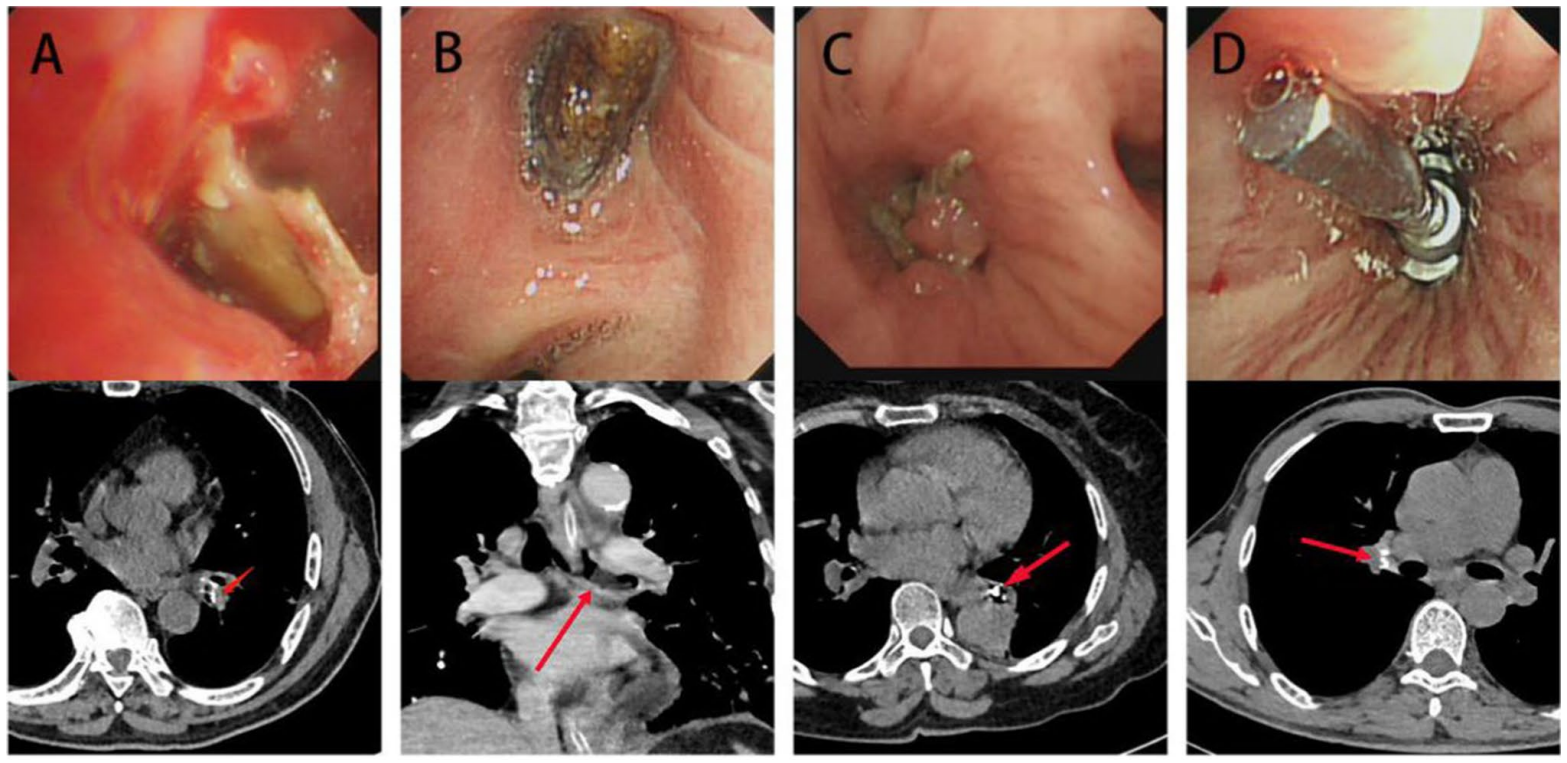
CT and bronchoscopy characteristics of different airway FBs. (A) A bone-like FB in the right inferior lobar bronchus. (B) A walnut in the left main bronchus. (C) A false tooth in the right inferior lobar bronchus. (D) A metallic FB^*^ in the left inferior lobar bronchus. FB, foreign body.
